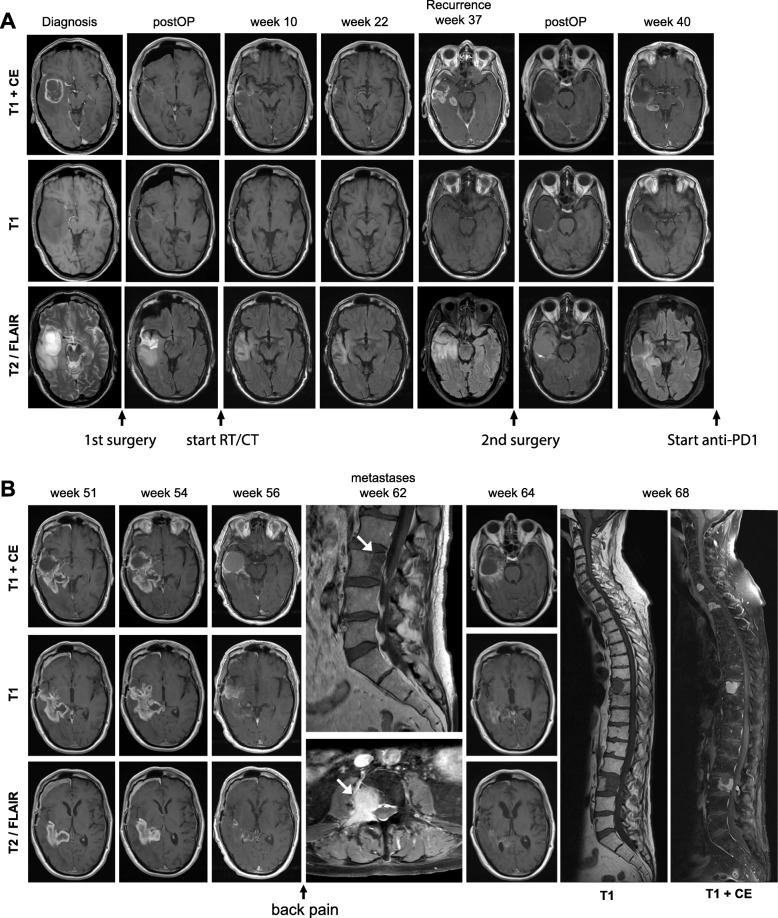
MR imaging and time-course. a MRI at various time-points during disease progression until anti-PD1 was administered. b Intracranial flare-up and remission after initiation of anti-PD1 treatment, and occurrence of multiple extracranial GBM metastasis while the intracranial tumours remained stable (week 64). White arrows show the intraosseous GBM metastases
